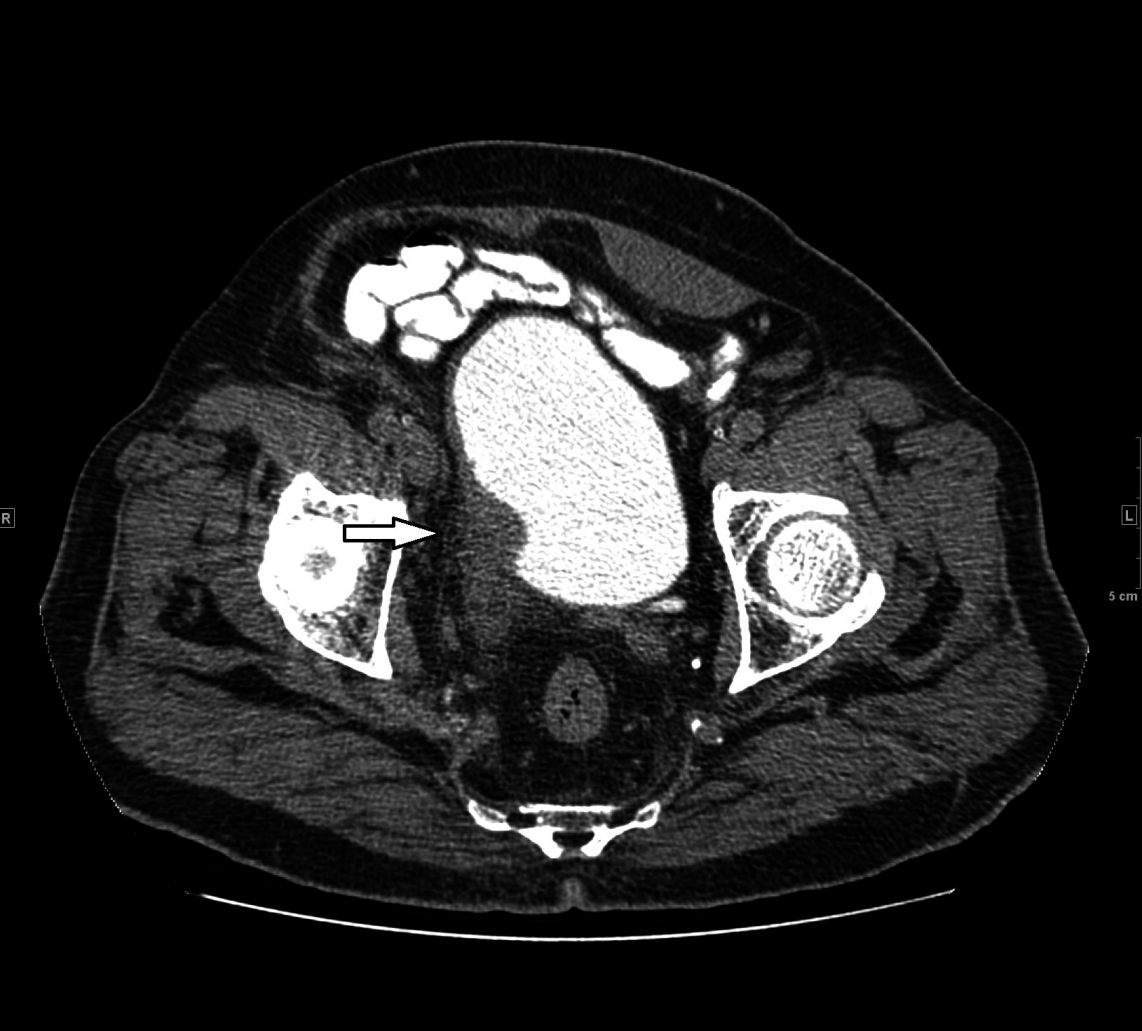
Delayed axial post-contrast computed tomography image showing irregular thickening of the right posterolateral bladder wall involving the ureterovesical junction
